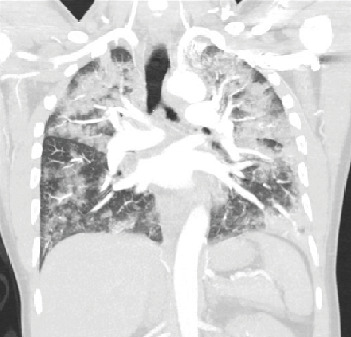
CT angiogram of the chest demonstrating diffuse multifocal ground-glass opacities with peripheral sparing and left pleural effusion.
